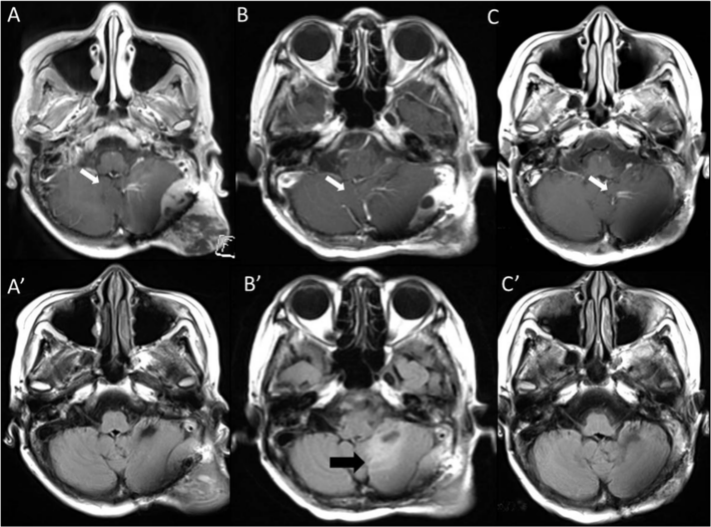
MRI of the patient’s brain before and after the BNCT. White arrows indicate a venous angioma, which was recognized incidentally and judged as a sectional standard of MRI. A: Gd-enhanced T1-weighted MRI of the brain 1 month before the BNCT. There was a subcutaneous and epidural tumor mass. B: Gd-enhanced T1-weighted MRI at 4 days after BNCT. The tumor mass was reduced. C: Gd-enhanced T1-weighted MRI of the brain 3 months after BNCT. The tumor mass was further reduced. A’: Fluid-attenuated inversion recovery (FLAIR) MRI of the brain 1 month before BNCT. B’: FLAIR MRI of the brain 4 days after BNCT. The tumor mass was reduced, but the edema had worsened. A black arrow indicates the cerebellar edema. C’: FLAIR MRI of the brain 3 months after BNCT. The tumor mass was further reduced, and the edema had disappeared.
